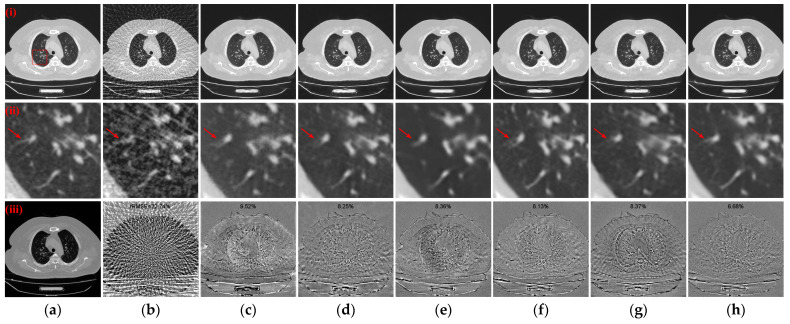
Qualitative comparative results of different models on the COVID-19-CT dataset with 64 projection views. (a) The reference image; (b) FBP; (c) MWNet; (d) DuDoTrans; (e) DDPTransformer; (f) MIST; (g) RegFormer; (h) Ours. (i)–(iii) are reconstructed CT images, enlarged ROIs, and absolute difference images, respectively. The display window for the reconstructed CT images and enlarged ROIs is [−1200, 300] HU; the display window for absolute difference images is [−180, 180] HU.
